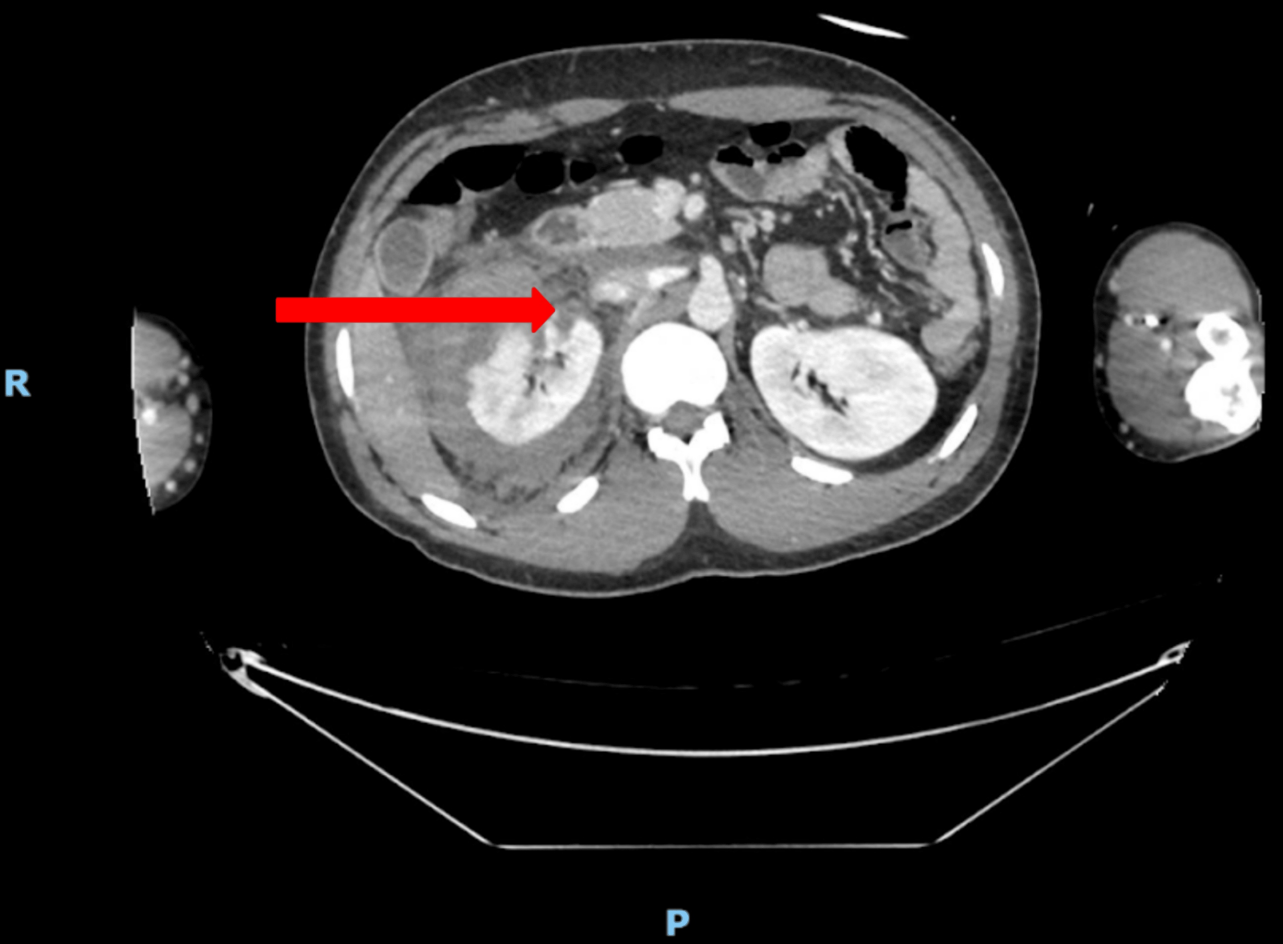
CT whole body trauma taken at hospital admission (day 0) The red arrow points to the disrupted renal pelvis in the shattered right kidney. The blue arrow points to the shattered right kidney. The letter R depicts the right-hand-side of the patient. The letter P depicts the posterior surface of the patient.
